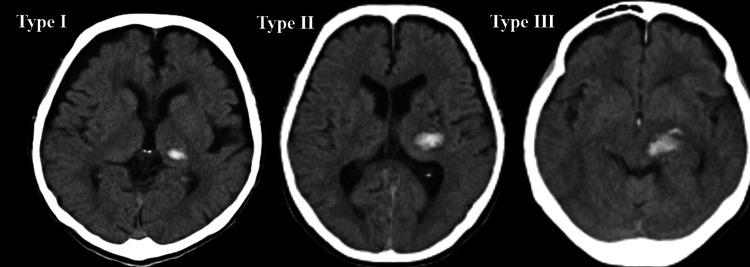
Classification of thalamic hemorrhage on computed tomography. Representative axial non-contrast computed tomography images of thalamic hemorrhage are shown. Type I was confined to the thalamus. Type II extended into the internal capsule. Type III reached the midbrain.
